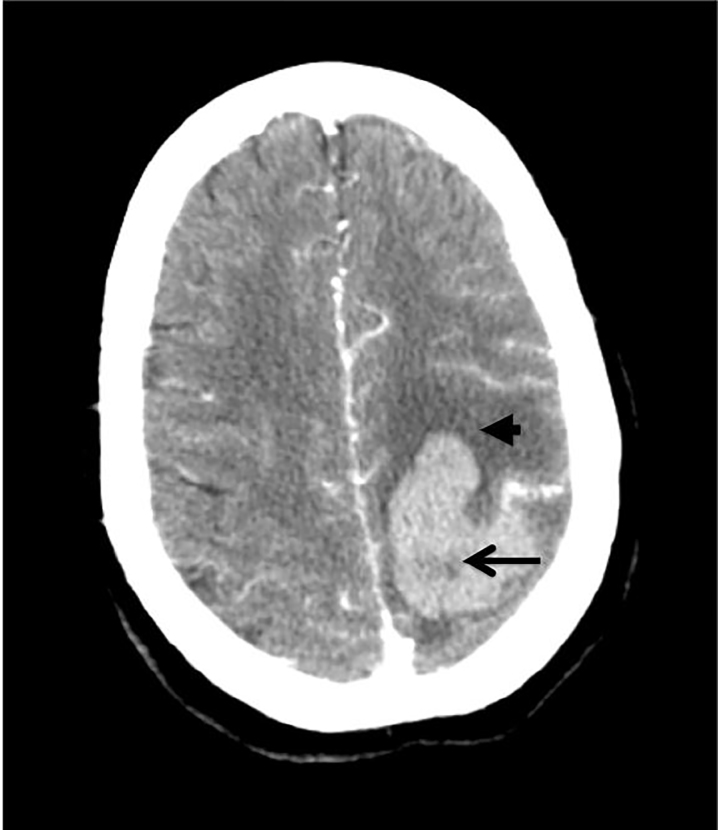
Computed tomographic angiography of the patient’s brain demonstrating left middle cerebral artery mycotic aneurysm with intracerebral hemorrhage (black arrow) and surrounding vasogenic edema (arrow head).
